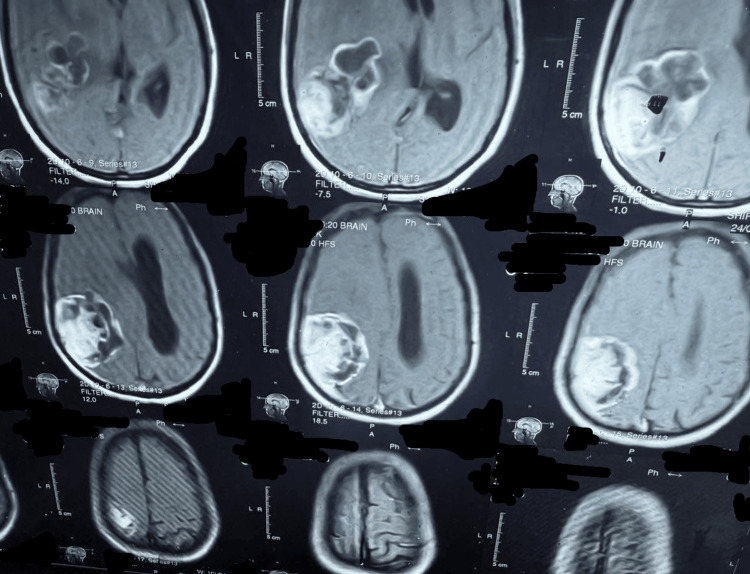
Axial MRI cuts demonstrating the superior-inferior and anterior-posterior tumor extension
